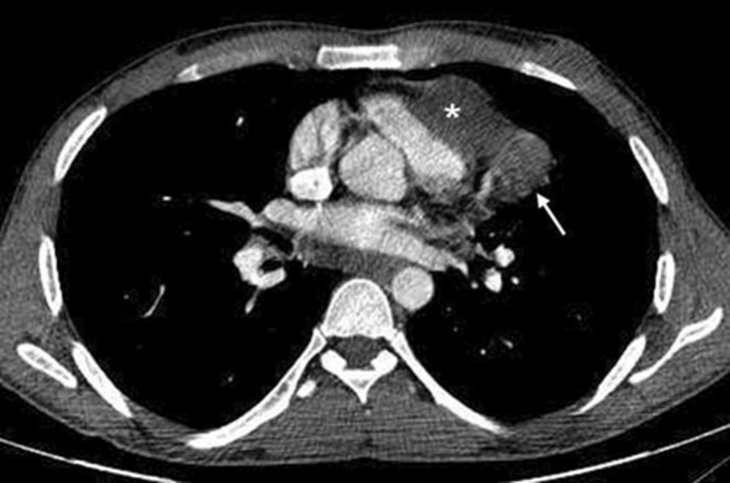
A 24-year-old male with Behçet's disease. Axial CT image shows ruptured pseudoaneurysm of the left anterior descending coronary artery (*white arrow*) with accompanying haematoma (*white asterisk*)
